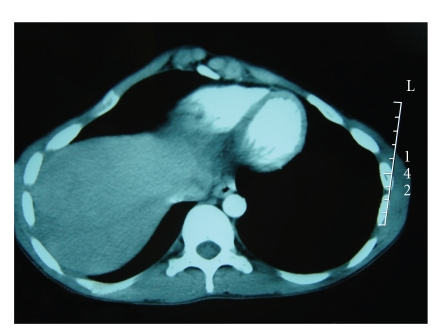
CT scan showing a decrease in tumor size which is evaluated to be 2 cm.
